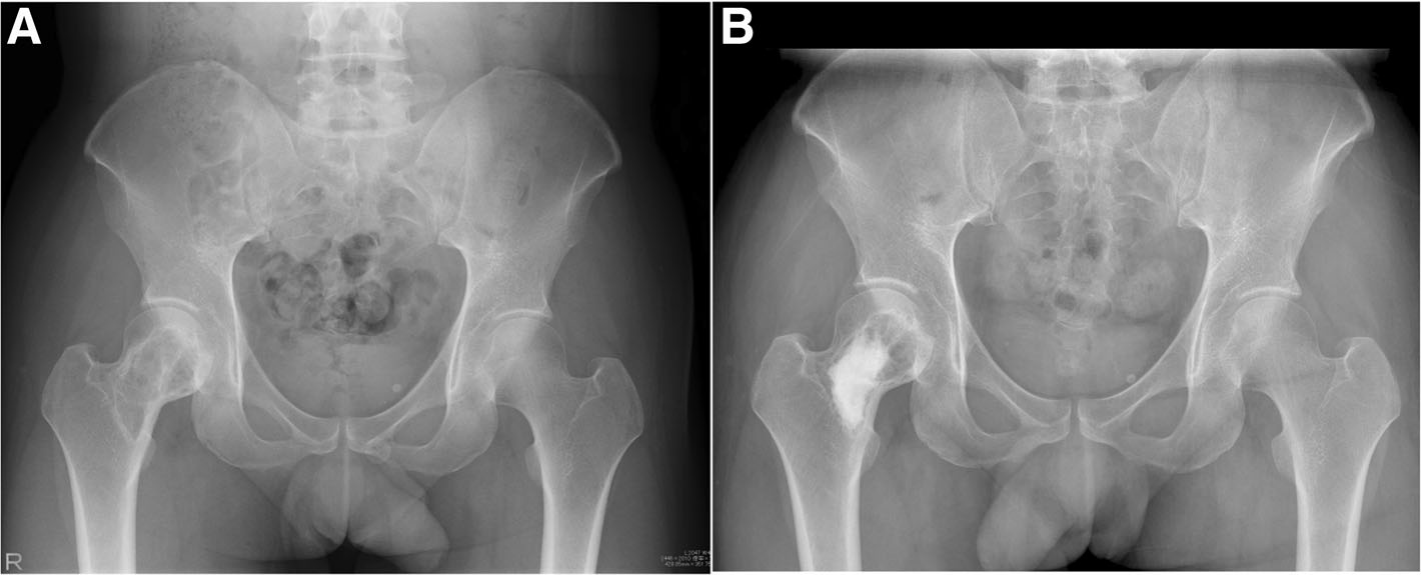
Patient 9: a AP radiograph showing proximal femoral lesion. b Postoperative AP radiograph at 48 weeks after plain ATP reconstruction showing no radiographic change of the lesion. Note the ATP reconstruction of only the lytic lesion, sparing nonosteolytic areas
